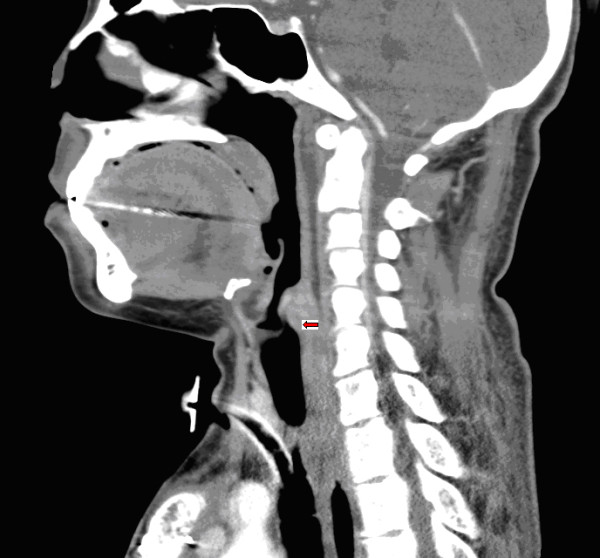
Sagittal and coronal view of the CT scan showing a short segment stenosis just above the tracheostomy.
